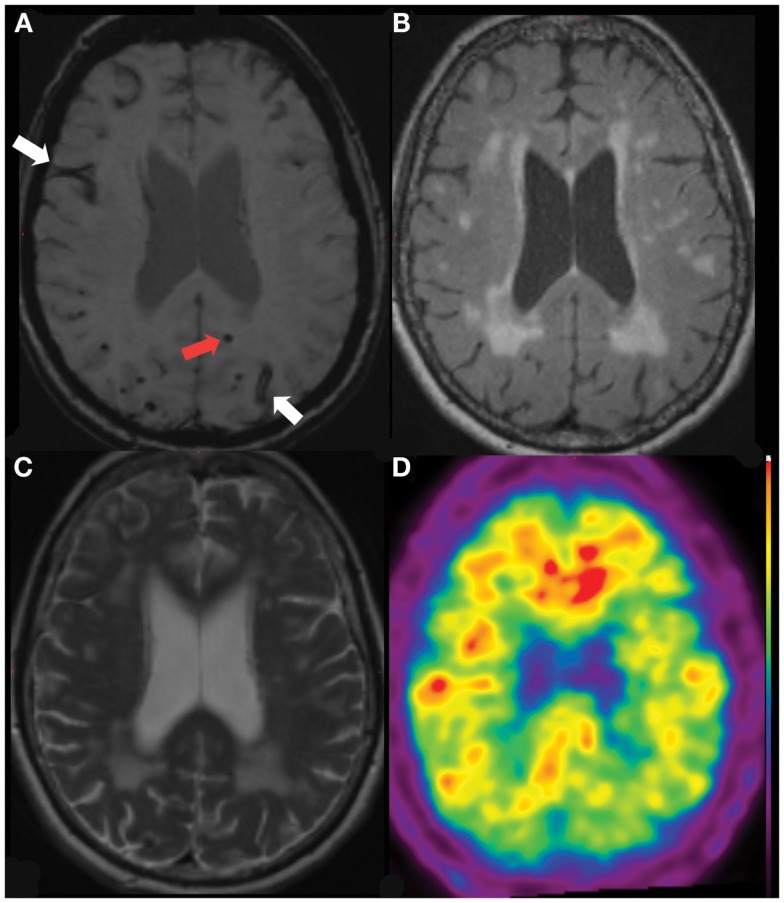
Lobar Microbleed (red arrow) and Superficial Hemosiderosis (white arrows) in a 66-year-old lady referred initially with amnestic MCI (MMSE 22/30, CDR 0.5, CDR-SOB 4.5), subsequently diagnosed with AD-type dementia. SWI image (A), with coregistered FLAIR (B), T2 (C) and 11C-PiB PET (D) images demonstrating severe deep white matter hyperintensities with elevated beta-amyloid burden (neocortical SUVR = 1.7).
